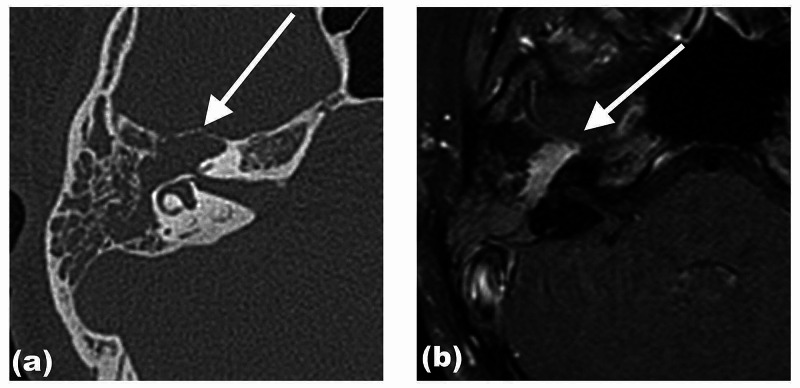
Axial view of HRCT of the right temporal bone (a) and contrast-enhanced T1-weighted (b) There is an expansion of the genu and tympanic segments of the right facial canal associated with bone dehiscence (a, white arrow). Note the enhancement of the facial nerve schwannoma, involving the genu and tympanic segments sparing the labyrinthine segment (b, white arrow). HRCT - high-resolution computed tomography
